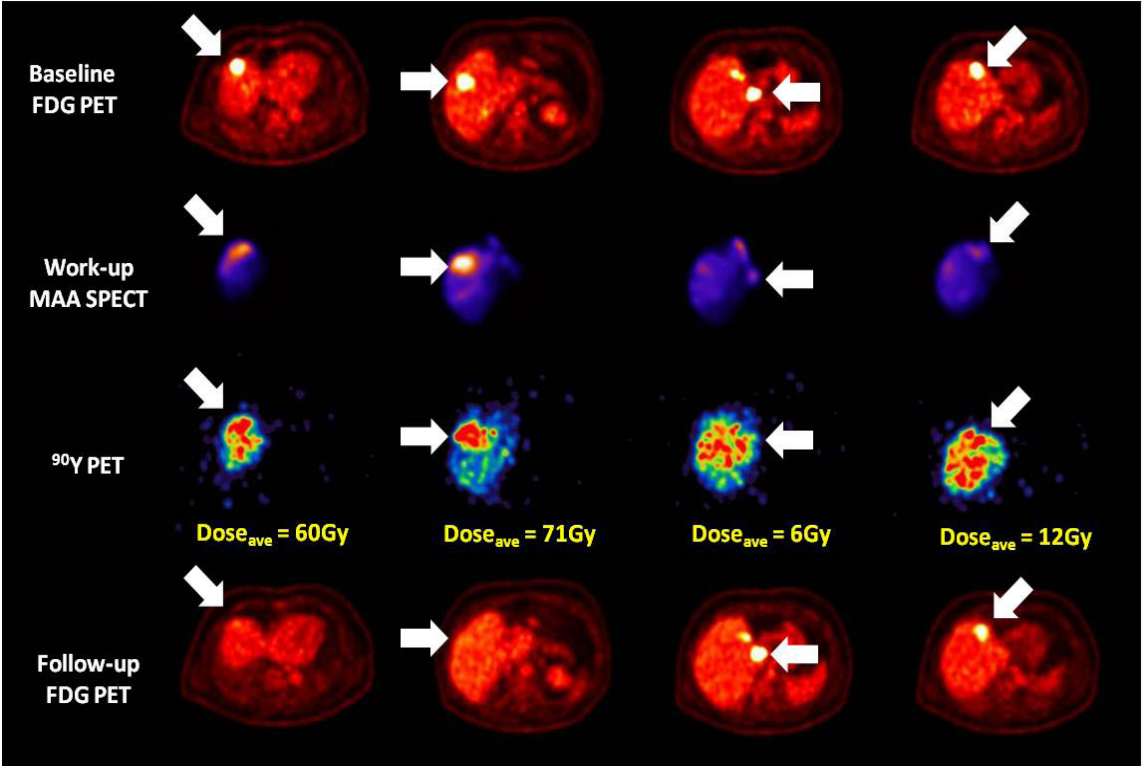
Direct comparison of transverse slices on the baseline FDG PET (upper row), work-up MAA SPECT (second row), ^90^Y PET derived dose map (third row), and 8 weeks follow-up FDG PET (bottom row) for each of the four lesions identified for SIRT. Lesions receiving an absorbed dose above the critical threshold can be seen to exhibit a complete response at the time of follow-up, whereas those receiving sub-threshold dosing exhibit stable disease
